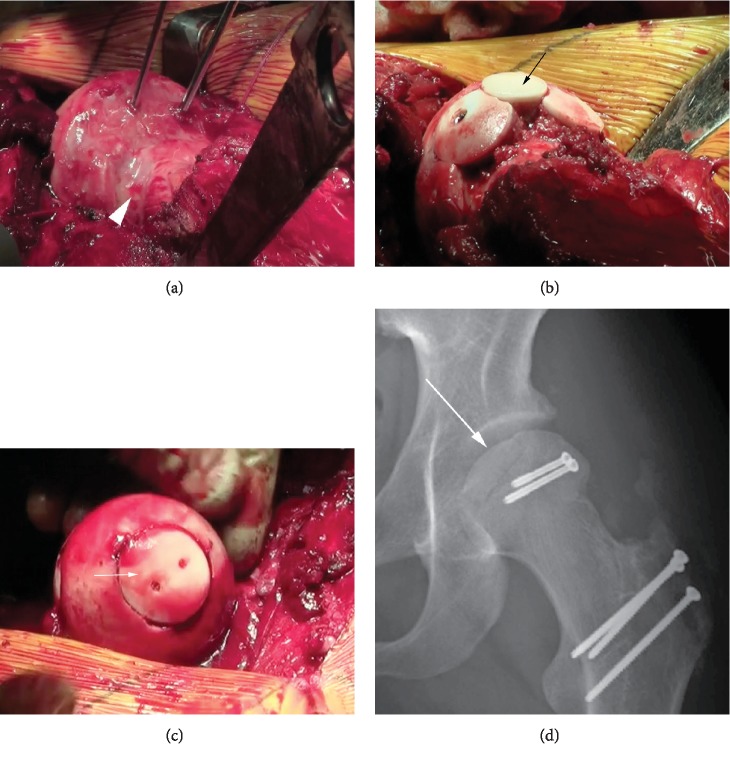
(a) Intraoperative photograph demonstrating guidepins placed in the lateral femoral head defect prior to coring for osteochondral allografting. The white arrowhead shows the anterior extent of the lateral femoral head lesion. (b) Intraoperative photograph demonstrating osteochondral allografts restoring the lateral contour of the femoral head at the side of the lateral femoral head defect. The central graft (black arrow) is in the process of being fully seated. (c) Intraoperative photograph of the femoral head apex with a 25 mm osteochondral allograft (white arrow) stabilized by two PLLA pins. (d) Immediate postoperative AP hip radiograph after femoral head osteochondral allografting through a trochanteric osteotomy with 2 metal screws holding the lateral femoral grafts in place. The apical femoral head graft is demonstrated by the white arrow.
